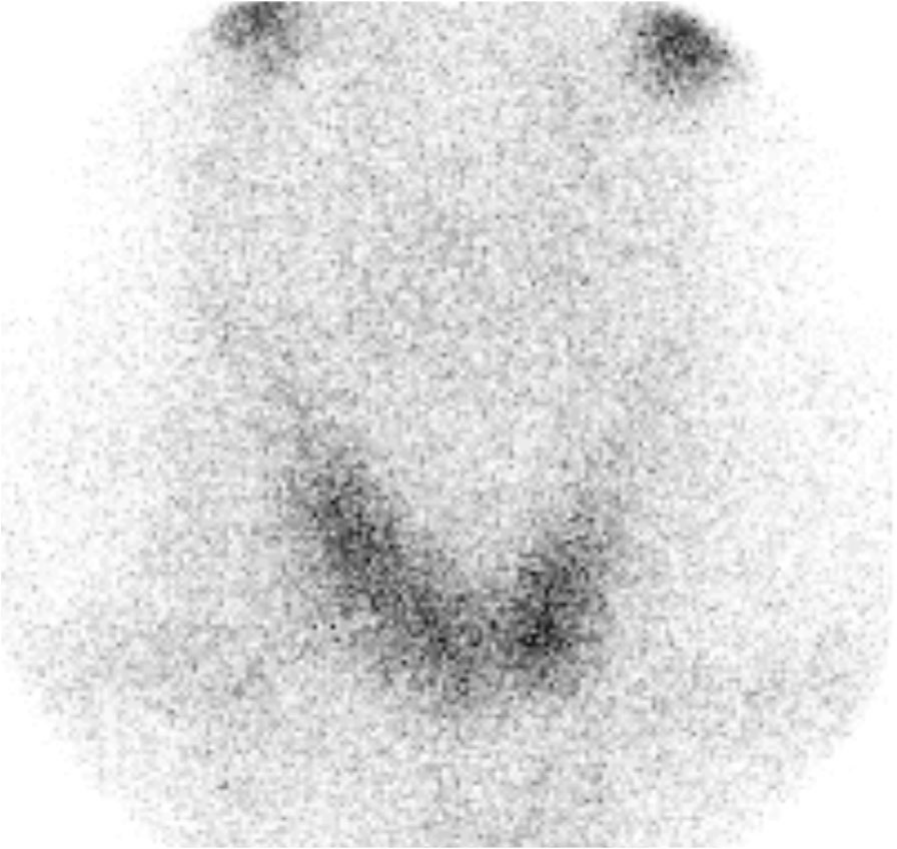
Thyroid scintigraphy showing irregular uptake throughout the thyroid gland
